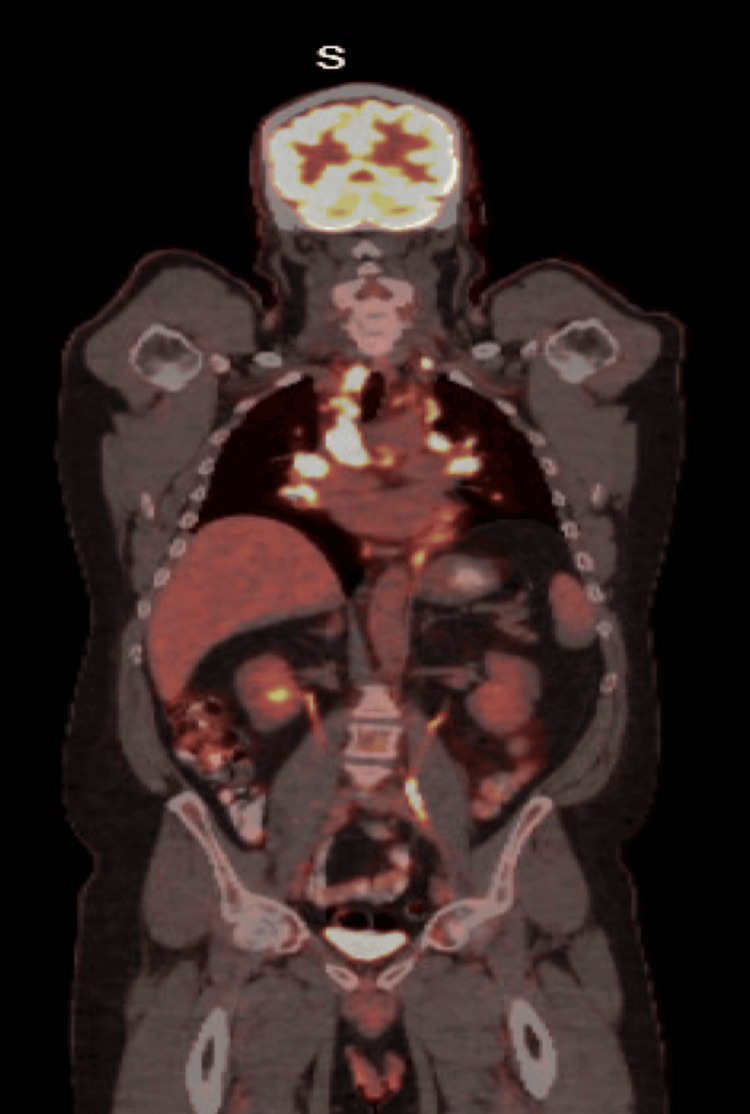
PET-CT scan highlighting FDG-avid supraclavicular, mediastinal, hilar, and pulmonary lymph nodes. FDG: fluorodeoxyglucose
